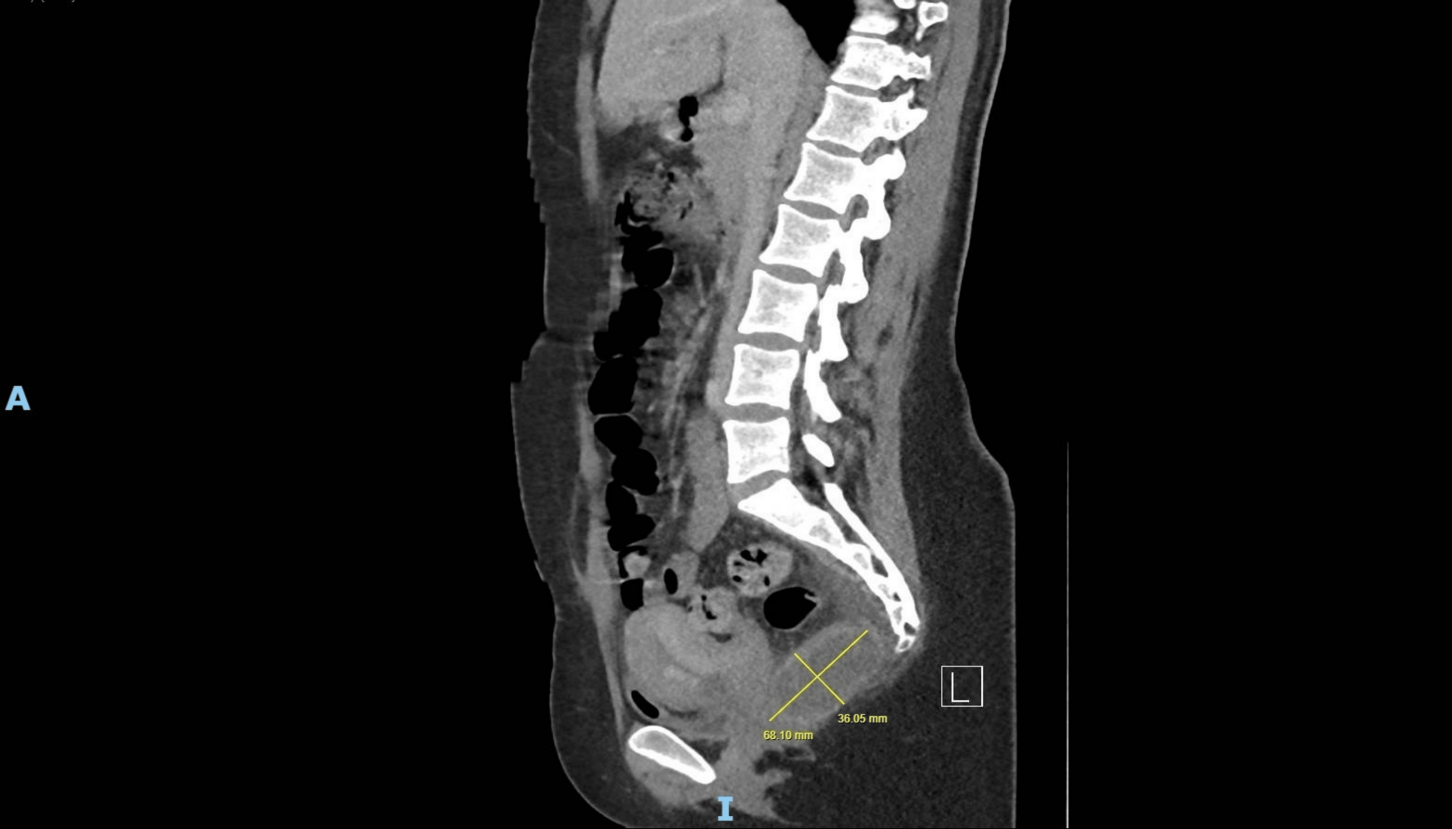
Sagittal CT pelvis (2020) showing pre-coccygeal lesion with peripheral enhancement and proximity to the rectum and coccyx
